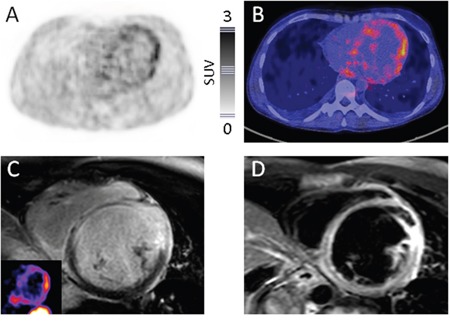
Display of a patient with active myocardial sarcoidosis Consistency of ^68^Ga-DOTATOC-PET and CMR Display of transaxial ^68^Ga-DOTATOC-PET (A., color bar indicating standardized uptake values), fused PET/CT B., short-axis late gadolinium enhanced (LGE) (C., insert: corresponding PET slice), and short-axis T2-weighted CMR D. slices in a 42-year-old woman (patient #8) with multi-organ sarcoidosis. PET presents enhanced tracer uptake in large portions of the left ventricle, highly consistent with cardiac involvement of sarcoidosis. CMR yields corresponding results. Of note, the patient experienced sustained ventricular tachyarrhythmia with subsequent cardiogenic shock five days after somatostatin-directed imaging requiring mechanical circulatory support with a percutaneous microaxial blood pump and veno-arterial extracorporeal membrane oxygenation.
